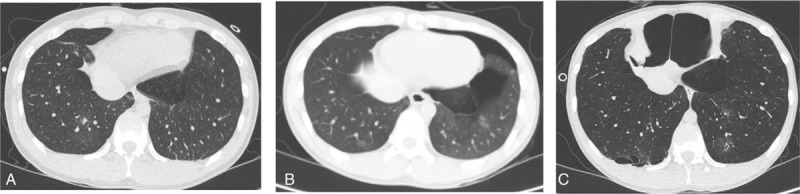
Serial chest CT scans. (A) HRCT scan taken 7 months before this admission (1st attack of pneumothorax-right side) shows nonspecific GGOs in both lower lobes. (B) Conventional CT scan taken 3 months before this admission (2nd attack of pneumothorax-left side) shows the GGOs with waxing and waning pattern compared with previous HRCT. (C) HRCT scan taken after bullectomy for the treatment of 3rd attack of pneumothorax (right side) shows more prominent GGOs and new cystic lesions (arrows) mainly in lower lobes and periphery. Hyperinflation with low attenuation in both lung parenchyma and the resultant interval change in the shape of thorax were also noted. CT = computed tomographic, GGO = ground glass opacity, HRCT = high resolution computed tomography.
